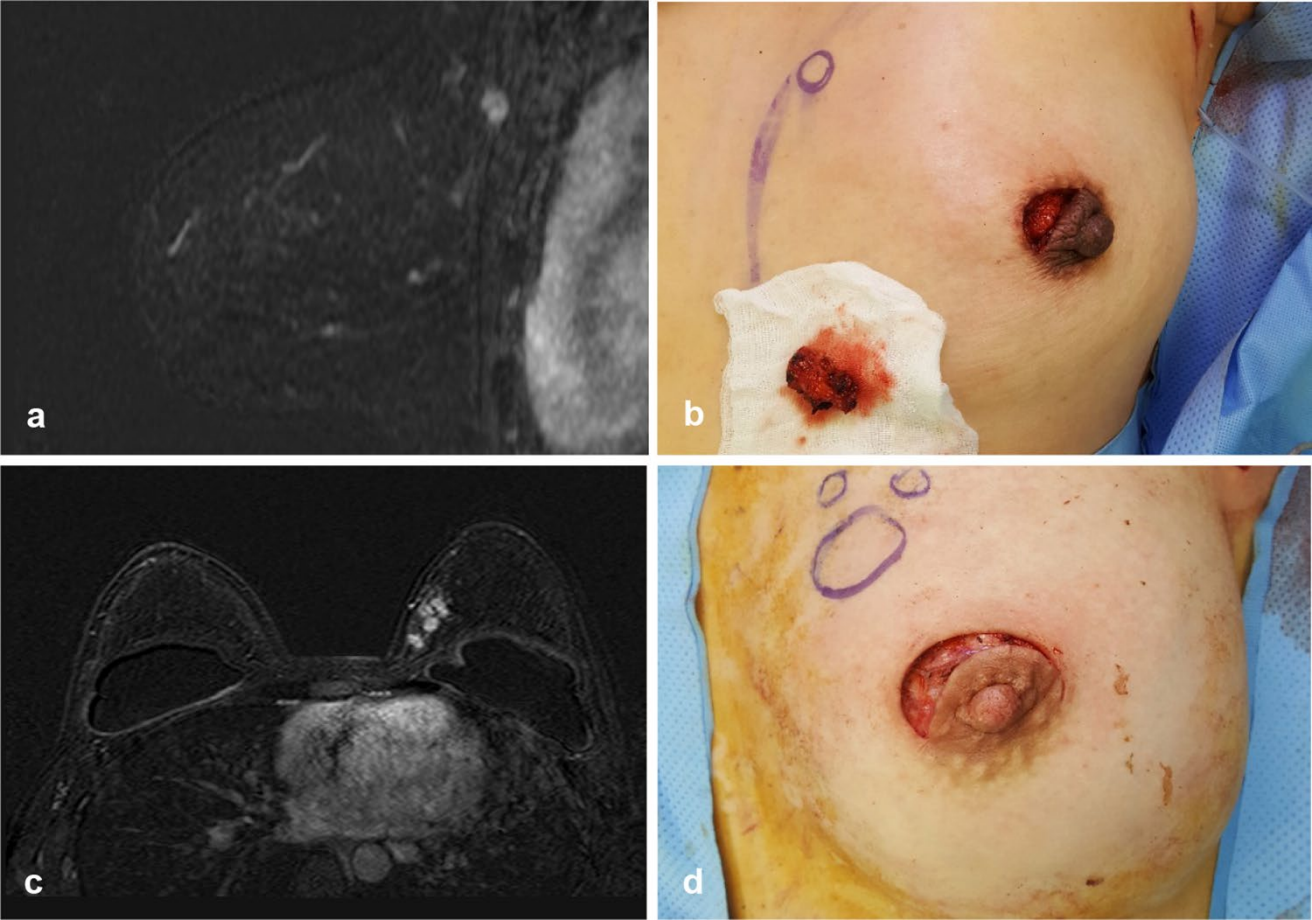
a A 48-year-old woman had a 1.4-cm cancer in the left upper inner quadrant, 12.3 cm from the edge of the nipple–areolar complex on magnetic resonance imaging (MRI). b Lumpectomy was performed through a periareolar incision. c Multifocal breast cancer was diagnosed in a 36-year-old woman with breast implants. The cancers were located in the upper inner quadrant of the left breast, 6.5 cm from the NAC on MRI. d The patient underwent lumpectomy via a periareolar incision and axillary dissection
